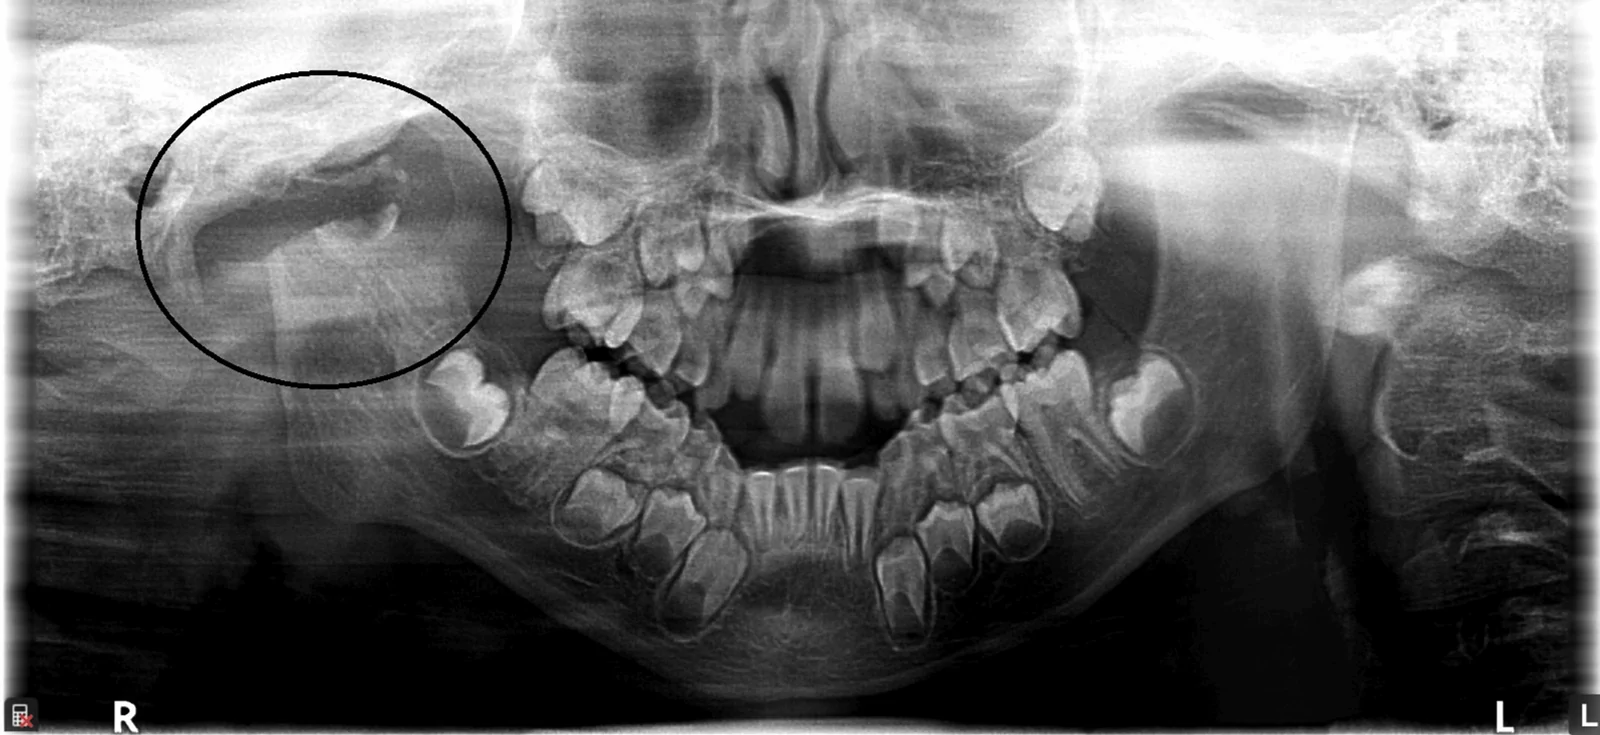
Postoperative orthopantomogram showing the surgically created gap in the right temporomandibular joint (black circle).
